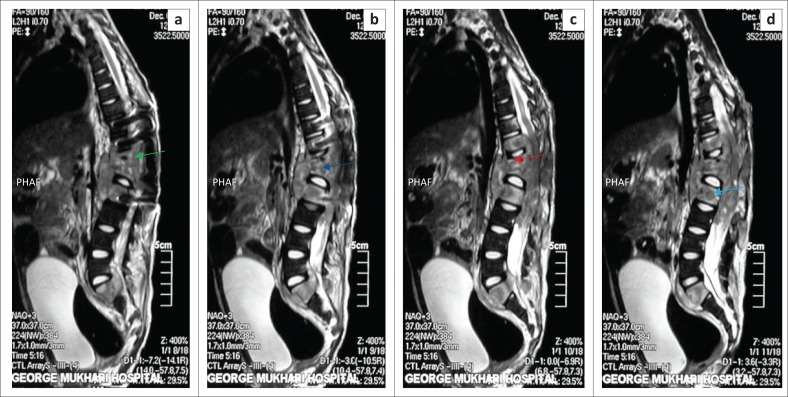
The magnetic resonance imaging of entire spine revealed (a = green arrow) an increased kyphosis, and (b = dark blue arrow) a paravertebral abscess. Furthermore, it demonstrated a complete vertebral destruction of lower and upper end plates of T12 (c = orange arrow) and L1 (d = light blue arrow).
